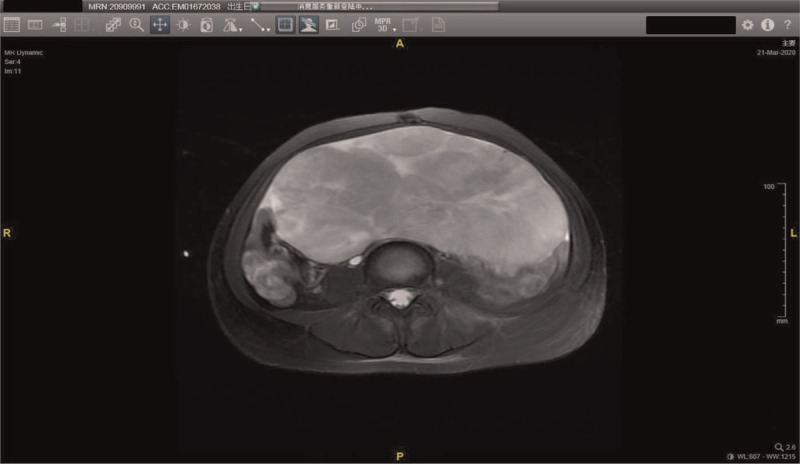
Contrast-enhanced MRI shows a huge abdominopelvic mass.
